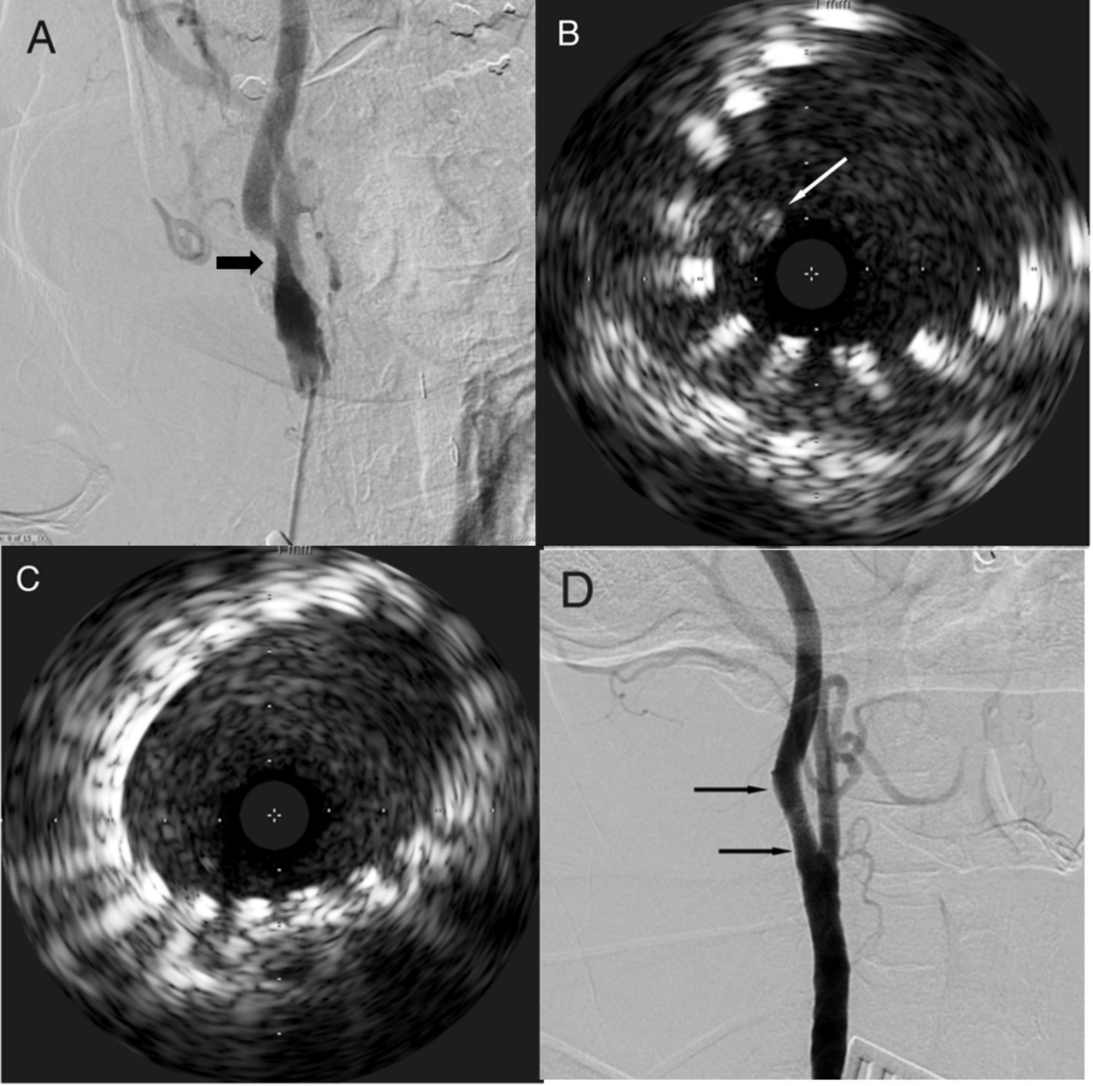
Digital subtraction carotid angiogram and intravascular ultrasound (IVUS) of Case 3 (A) Lateral projection diagnostic angiogram confirming severe stenosis (arrow) in the internal carotid artery just distal to the bifurcation. (B) Poststenting intravascular ultrasound (IVUS) image demonstrating thrombus inside the stent (arrow). (C) Final IVUS image after second stent deployment and balloon angioplasty demonstrating resolution of thrombus. (D) Final lateral projection angiogram demonstrating improved vessel caliber (arrows).
